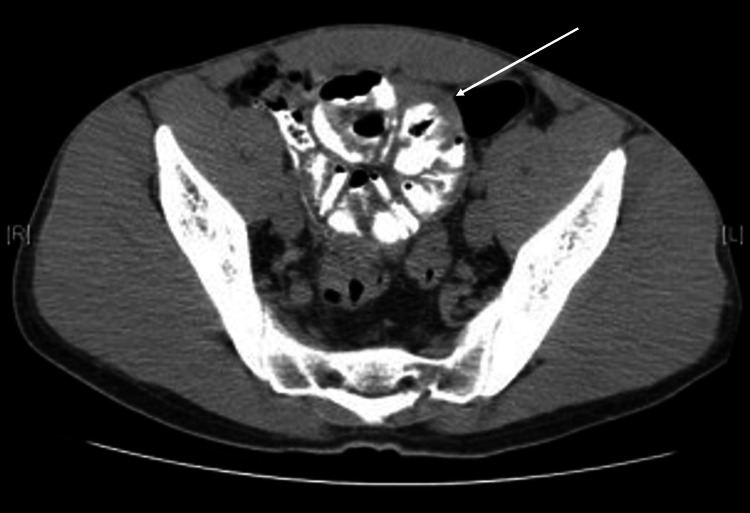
Coronal sections of abdominal CT images showed a part of small bowel embedded within a thin-walled fluid-filled sac-like structure.
